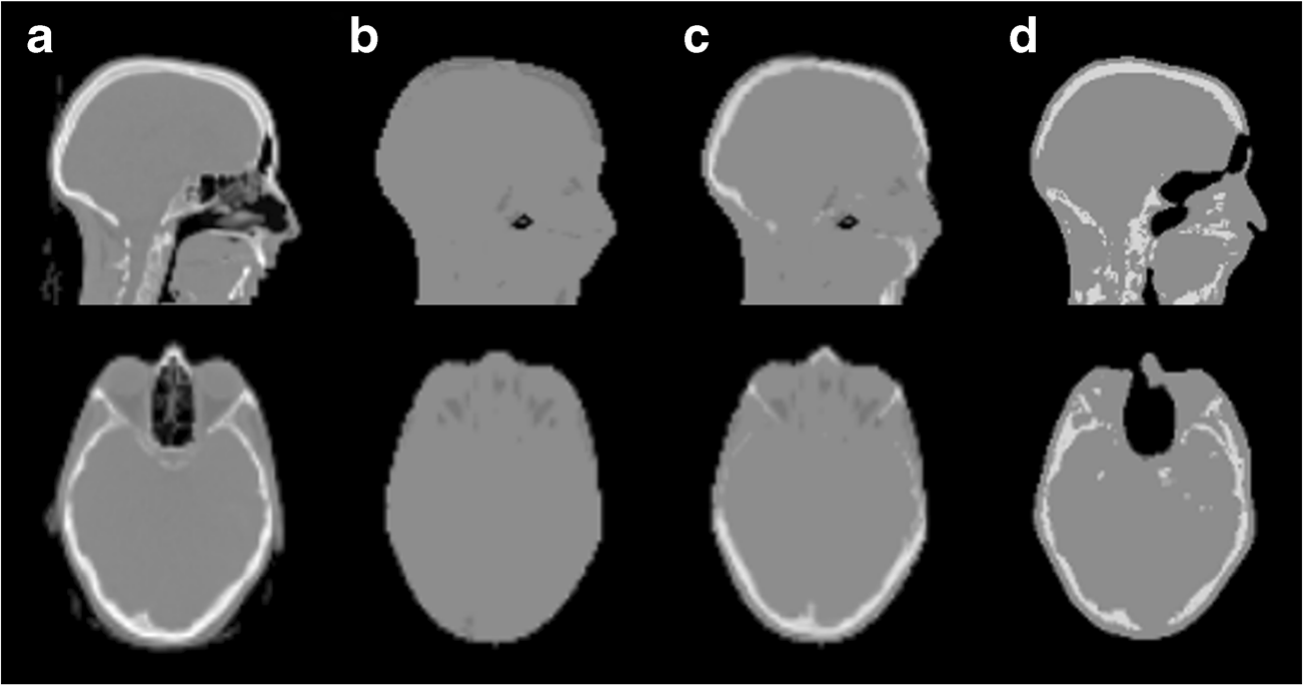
Example of the used MR-AC methods compared to CT-AC. a CT-AC. b Dixon-AC. c Model-based AC. d UTE AC. Image shows the same sagittal and axial slices of one patient
